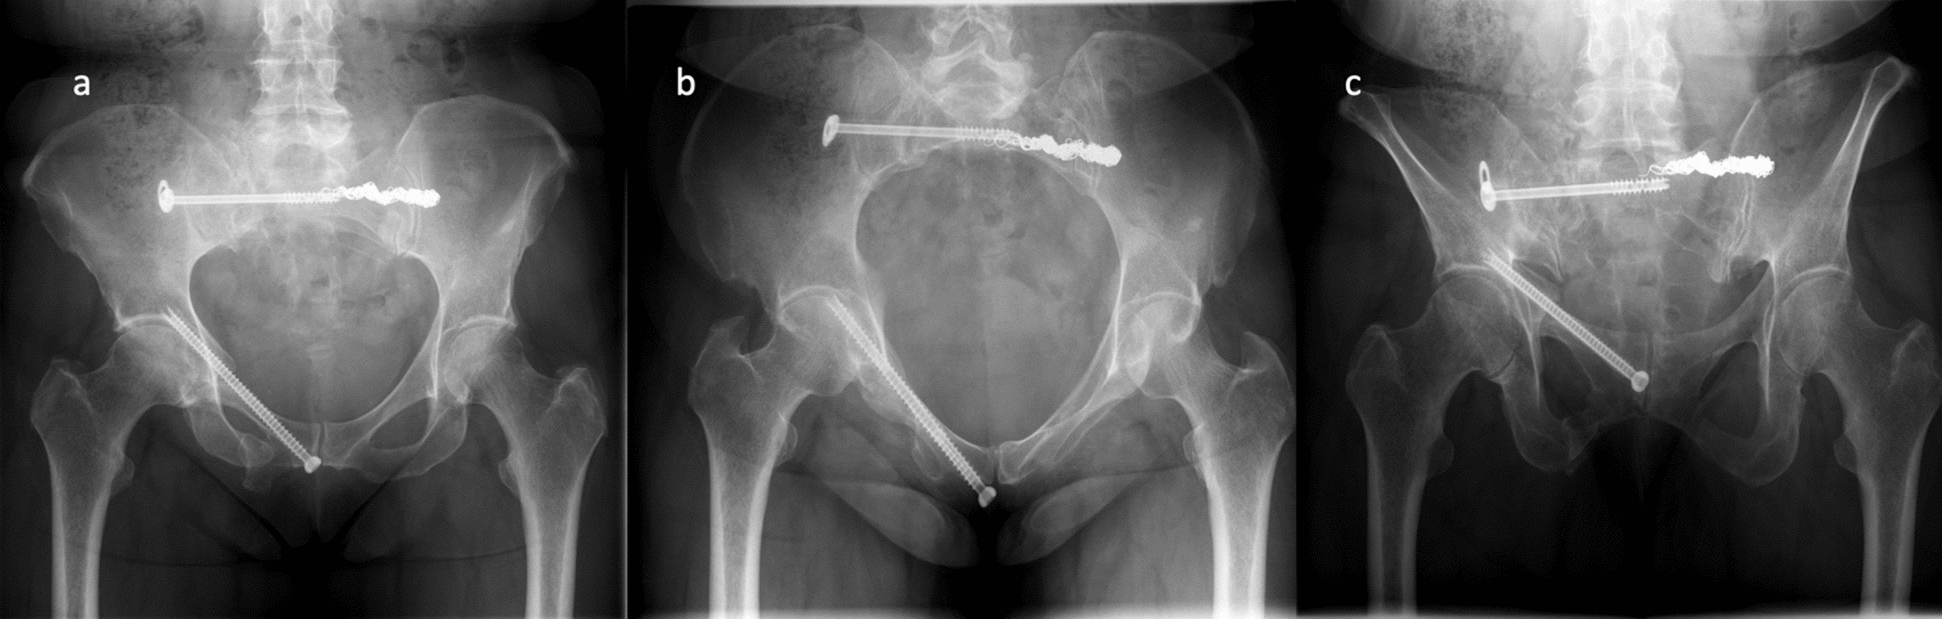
Nine-month follow-up X-rays, showing a pelvic X-ray a, as well as inlet b and outlet c view
